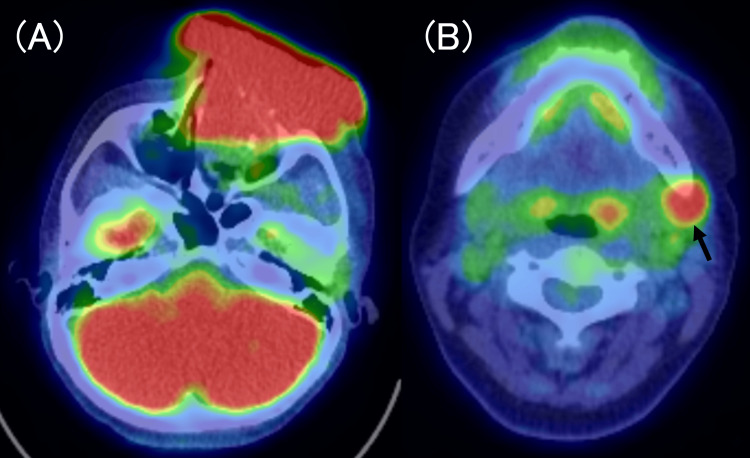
FDG-PET/CT scans. Elevated glycolytic activity is observed at the tumor site (A) and left submandibular lymph node (black arrow) (B) FDG-PET: fluorodeoxyglucose positron-emission tomography; CT: computed tomography
